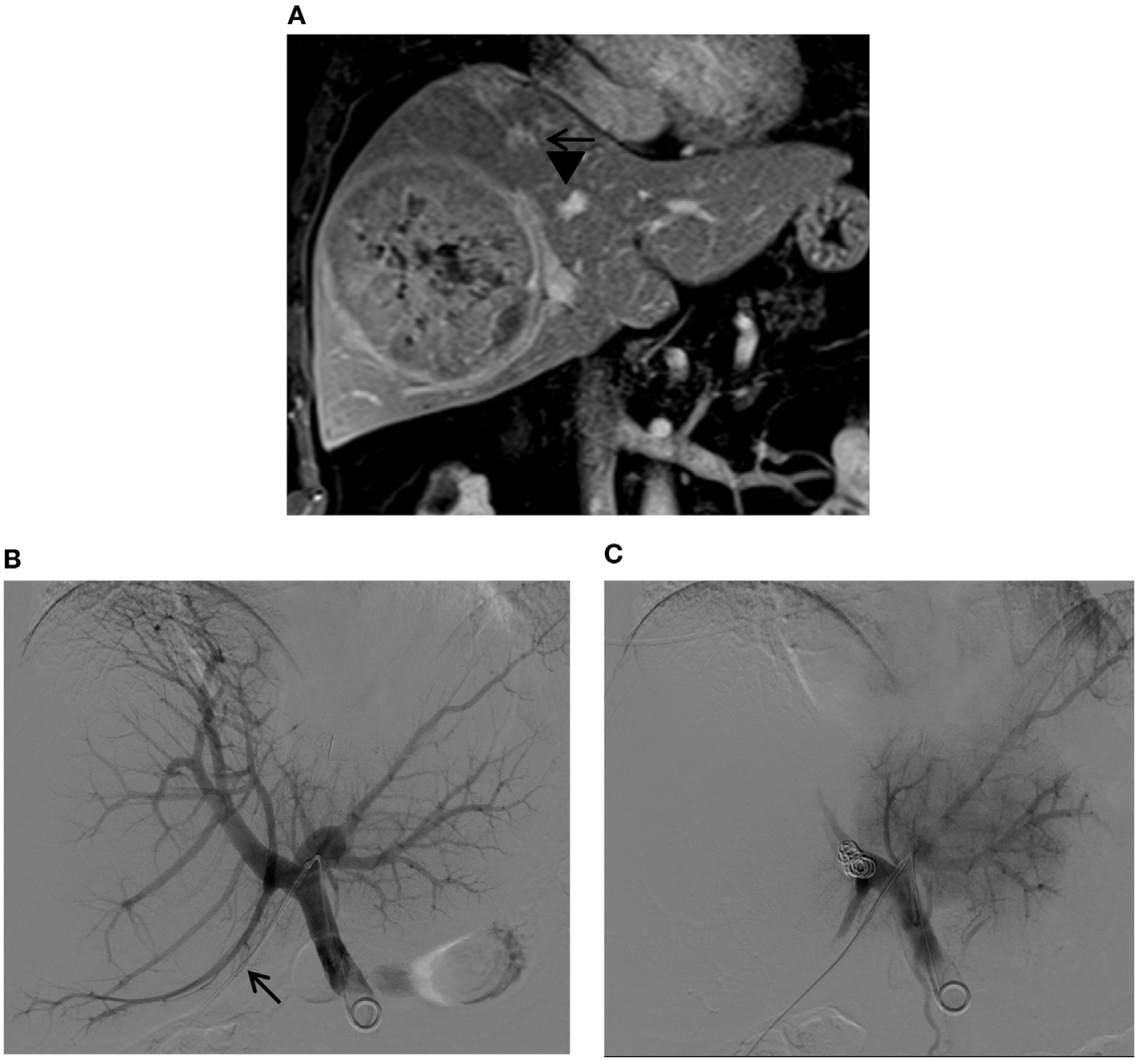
Right PVE *via* a contralateral approach in a patient with a large hepatocellular carcinoma. (A) Contrast-enhanced T1-weighted coronal MR images show a large tumor in the right liver. Marked distortions of the anterior (arrows) and posterior (arrowheads) branches of the right portal vein caused by the tumor are noted. The patient was referred for right PVE prior to a right hepatectomy. An ipsilateral approach was initially planned; however, no portal branches were identified for safe access within the right liver during the procedure. Access to the Sg4 portal vein branch was then obtained with a 22-gauge Chiba needle (not shown). (B) Flush portogram shows a 5-Fr pigtail catheter placed within the main portal vein through a 6-Fr sheath (arrow). (C) Final portogram shows successful occlusion of the right portal vein branches, with preserved blood flow to the left portal vein branches.
